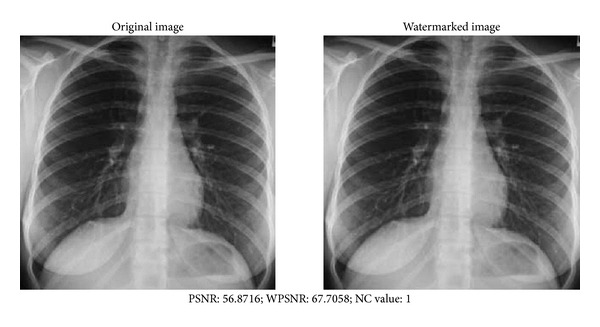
Original and watermarked Radiographic images.
